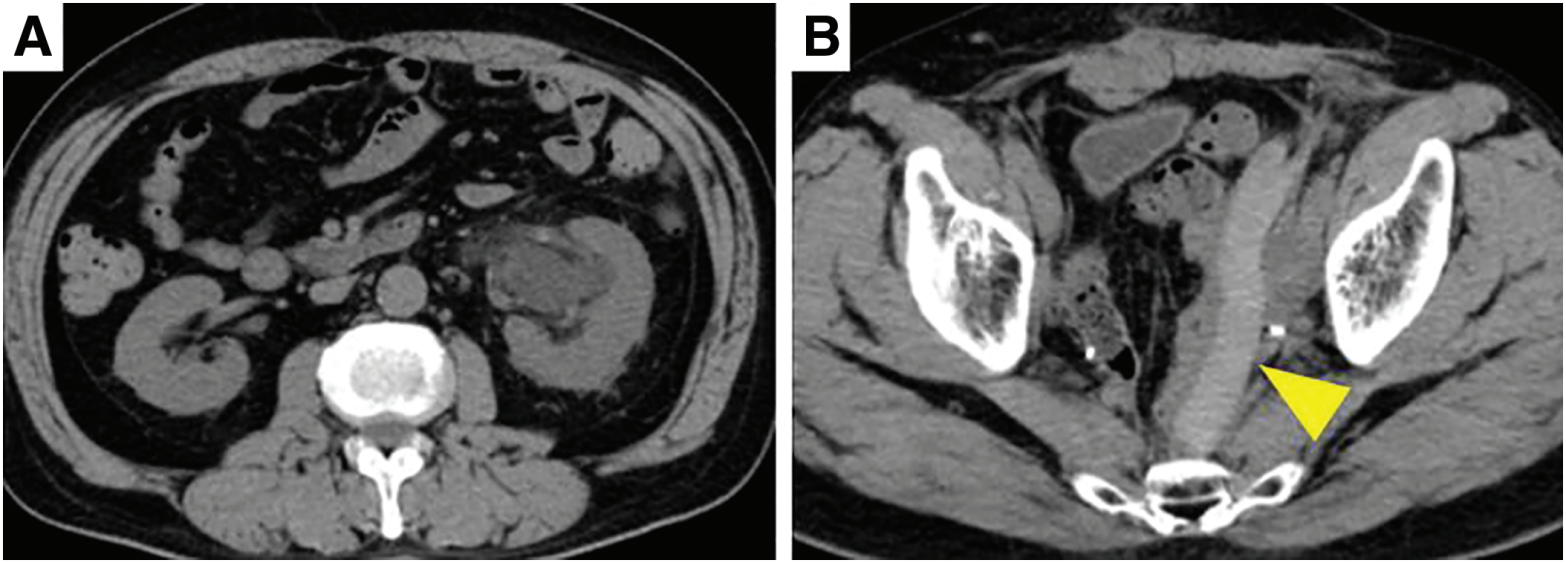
Plain CT image 7 days after PGA spacer placement. Left hydronephrosis was observed (A), and the cause was thought to be ureteral compression by the PGA spacer (arrowhead) (B). PGA, polyglycolic acid
